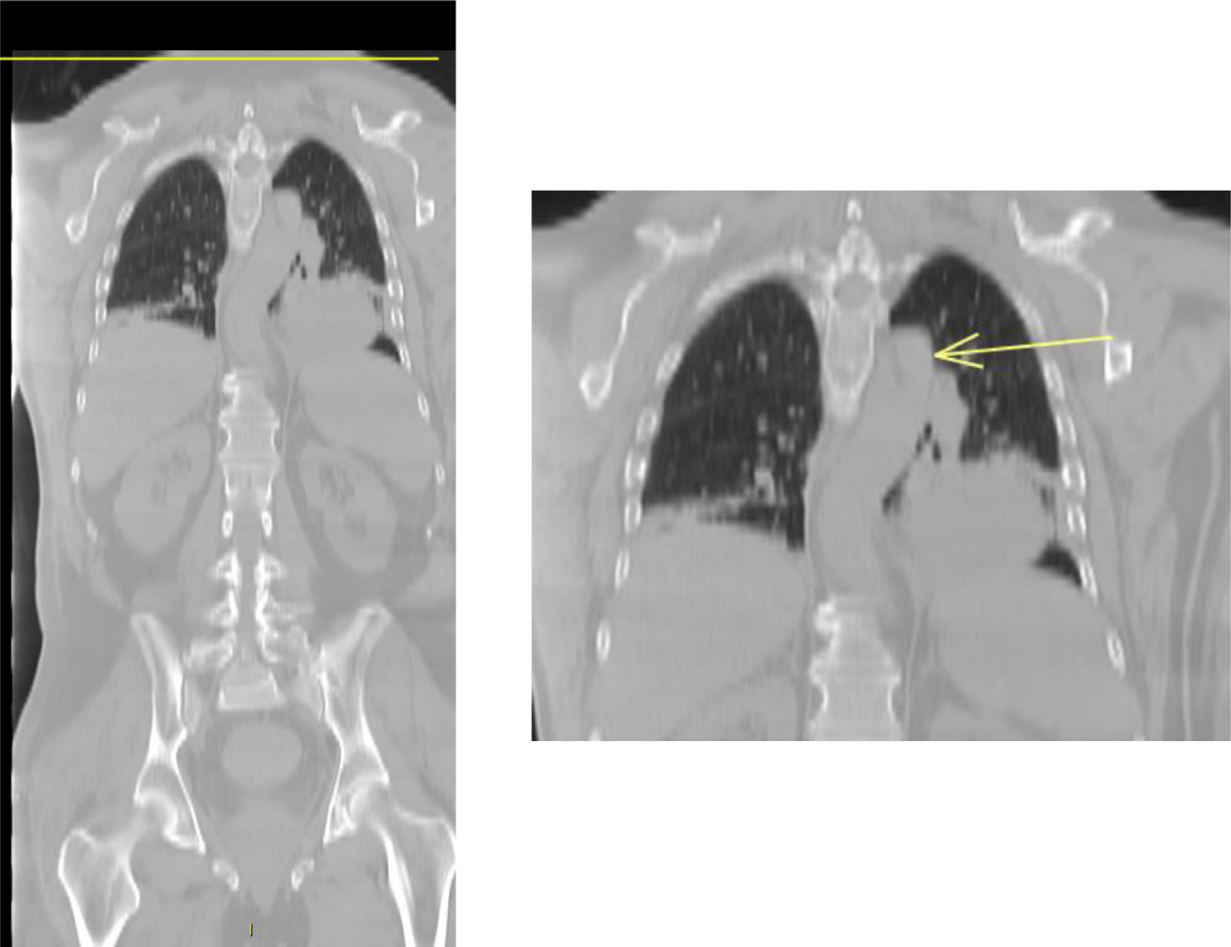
(A, B) Basal Chest High Resolution Computed Tomography (HRCT); coronal MPR reconstructions. HRCT had identified in the basal areas of both lungs the presence of thickenings with a ``ground glass'' pattern, and areas of interstitial consolidations and thickenings. There were also the presence of a minimal pleural effusion, and a lot of pericardial effusion. HRCT had also identified the presence, in basal conditions, of a thin intraluminal hypodense line in the thoracic aorta (yellow arrow), which raised the clinical suspicion of an aortic dissection.
